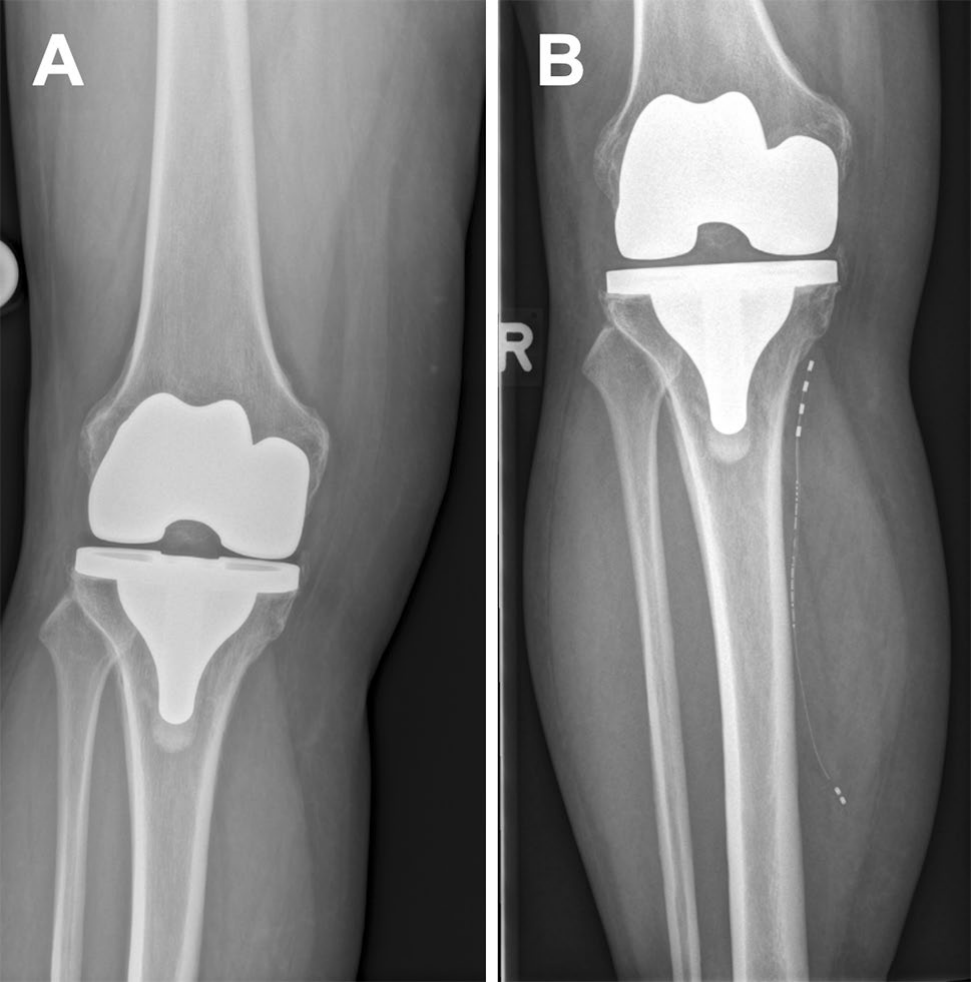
This is a 78-year-old subject suffering from chronic knee pain after total knee arthroplasty due to osteoarthritis. (A) Preoperative X-ray. (B) Postoperative x-ray with saphenous stimulation system in-situ.
